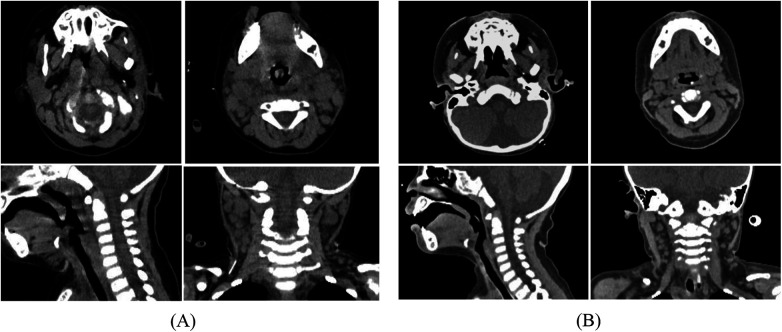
(A): Neck CT at admission shows extensive swelling and thickening of the nasopharyngeal, oropharyngeal, and laryngeal walls with narrowing of the laryngeal cavity; multiple enlarged lymph nodes in bilateral neck regions II, III, and IB with blurred surrounding fat planes. (B): Follow-up cervical CT scan on hospital day 7 showing marked reduction in the size of cervical and submandibular lymph nodes and resolution of parapharyngeal and retropharyngeal swelling.
